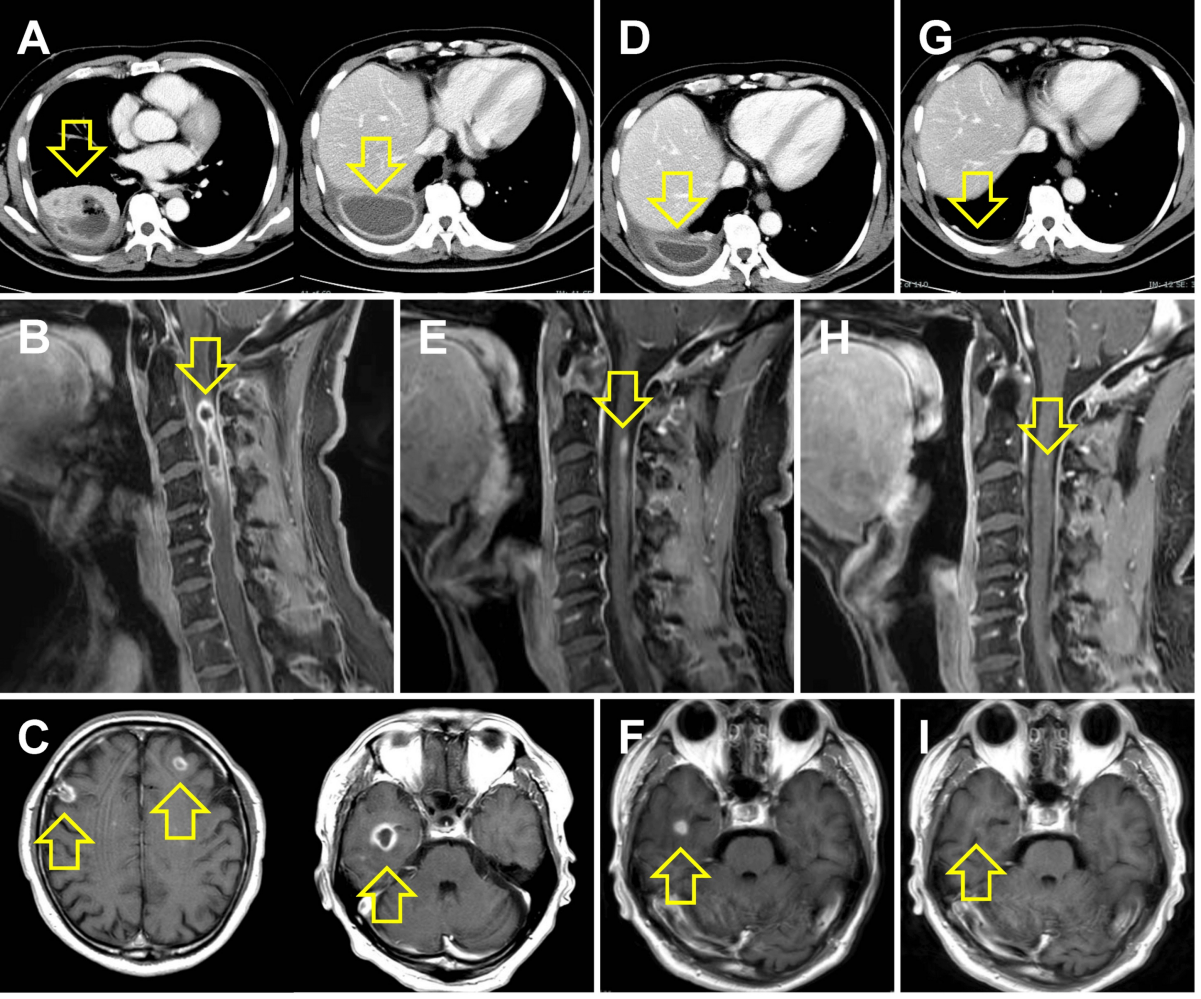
Imaging findings (A) Initial chest computed tomography (CT) scans obtained at admission showing an indistinct demarcation between the right lower lobe and right pleural effusion, with air presence within the effusion, and a segmental pleural effusion characterized by thick septations. (B) Spinal sagittal contrast-enhanced T1-weighted (CE-T1WI) magnetic resonance imaging (MRI) obtained at admission reveals an occupying lesion with peripheral contrast enhancement in the C2-4 region. (C) Brain axial CE-T1WI MRI indicates ring-enhancing lesions located in both the frontal lobes and the right temporal lobe. (D, G) Subsequent CT demonstrated a significant reduction in the size of the latter lesion at (D) 1 month and (G) 4 months following treatment initiation. (E, F, H, I) Subsequent MRI indicated a marked decrease in the size of these lesions at 1 month (E, F) and 4 months (H, I) following treatment initiation.
